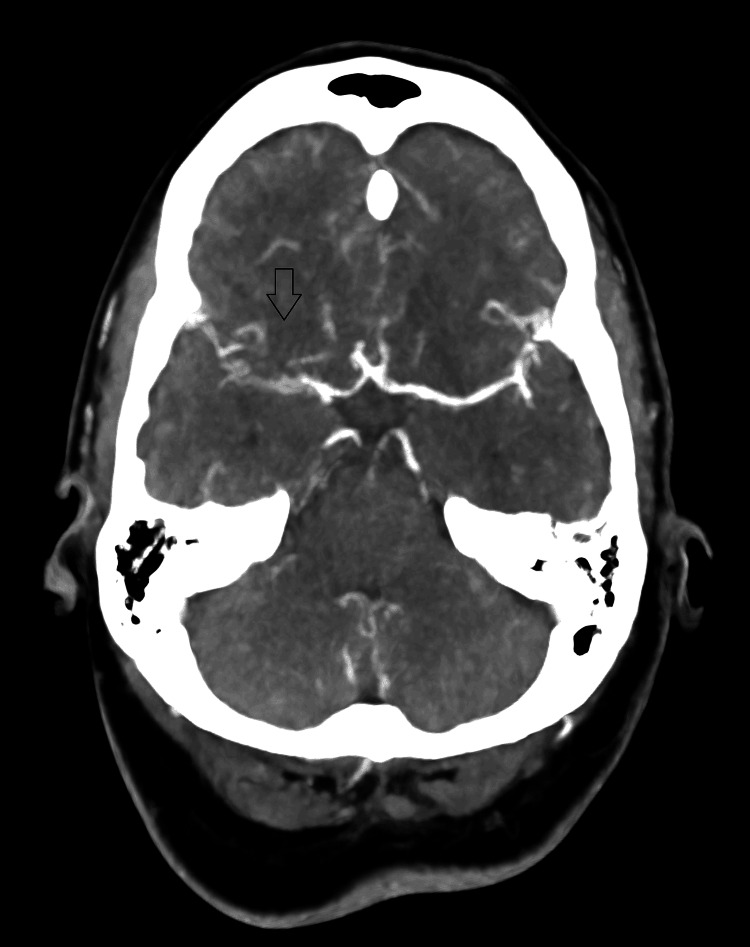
Axial CT angiography of the brain Axial slice from a CT angiography of the brain demonstrating reduced opacification of the right MCA M1 segment (black arrow) compared to the contralateral side. CT: computed tomography; MCA: middle cerebral artery
